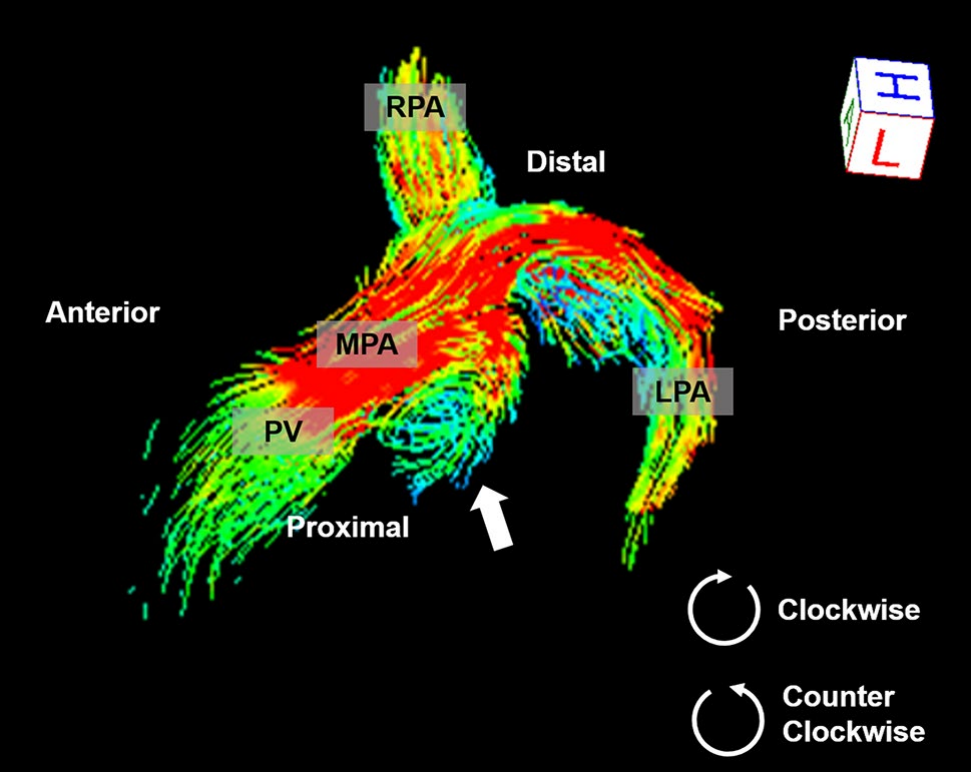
A 14-year-old boy with repaired Tetralogy of Fallot. Streamline imaging by 4D flow magnetic resonance imaging visualizes the pulmonary arteries. Clockwise vortices are seen at the posterior portion of the proximal main pulmonary artery when viewed from the patient’s left (arrow). Flow visualization was performed using 4D flow postprocessor software (iT Flow; Cardioflow Design. Inc., Tokyo, Japan). *MPA* main pulmonary artery, *LPA* left pulmonary artery, *RPA* right pulmonary artery.
